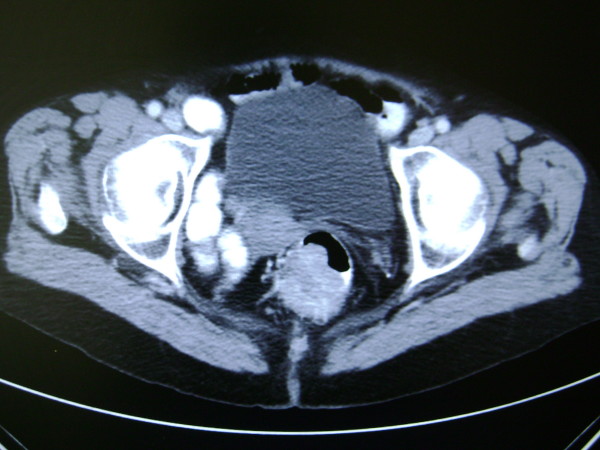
Pre-operative CT Abdomen-Pelvis image showing polypoid rectal lesion extending into the lumen.
